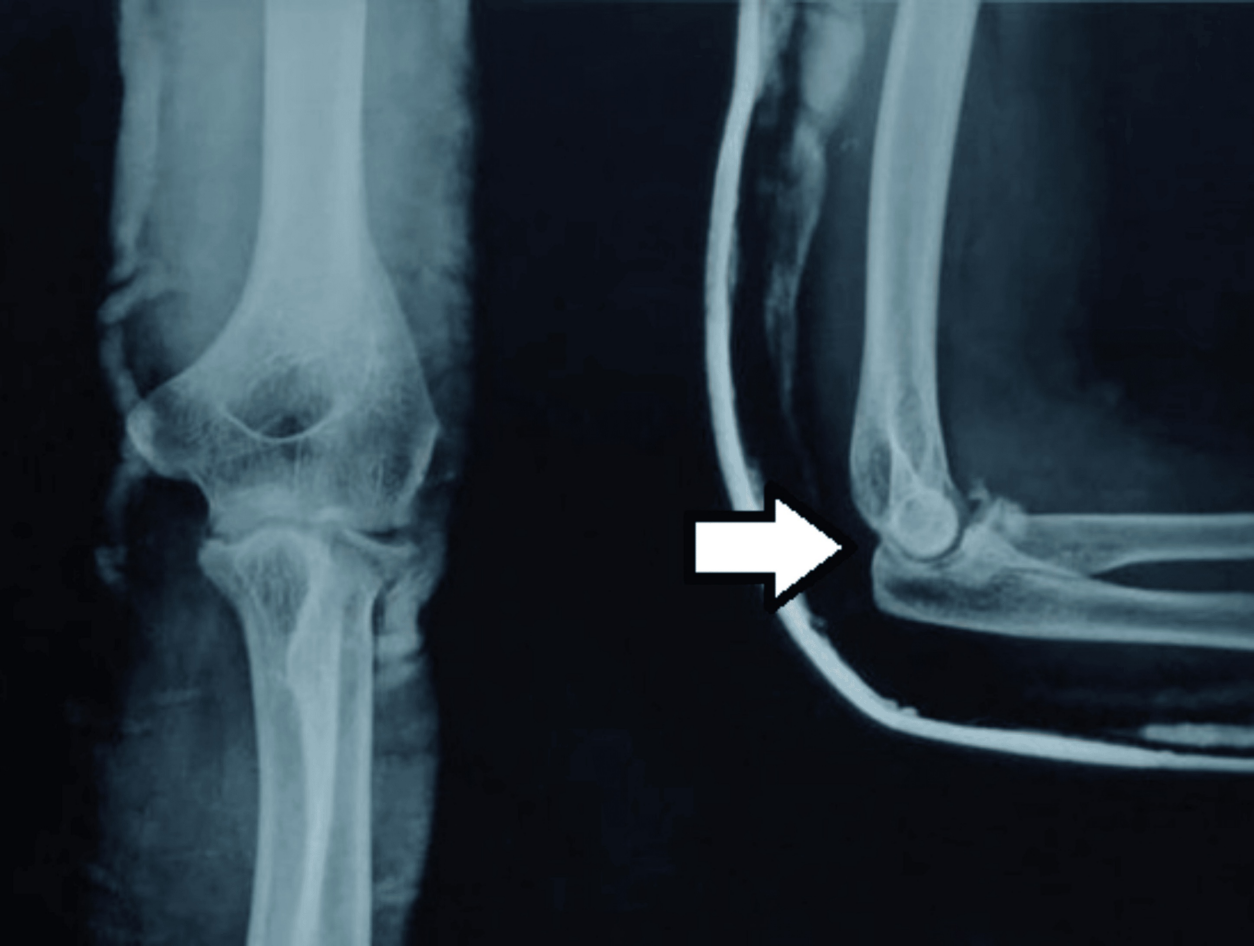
Postreduction AP and lateral radiographs of left elbow joint depicting reduction of elbow dislocation (white arrow) AP: Anteroposterior
